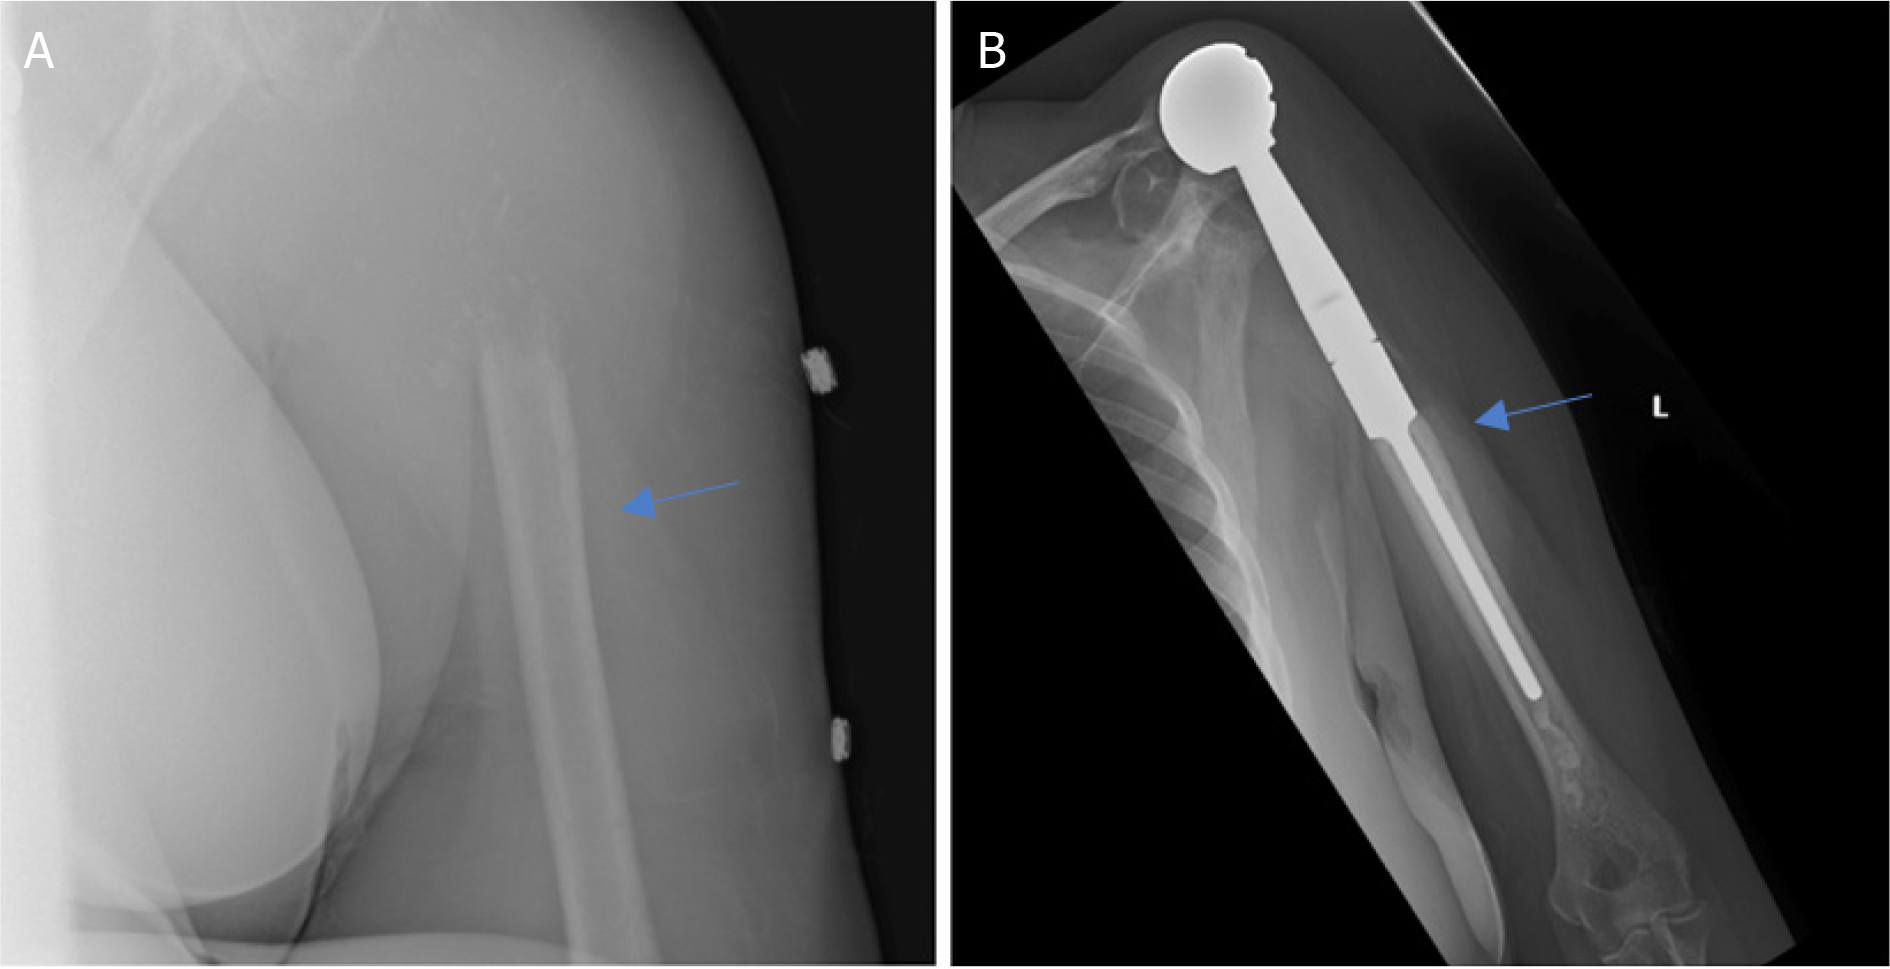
Anteroposterior radiographs of a 61-year-old female with: multiple myeloma of the proximal humerus preoperatively (A); five years after wide excision and proximal endoprosthesis reconstruction (B). Blue arrow notes the junction between the prosthesis and native bone.
